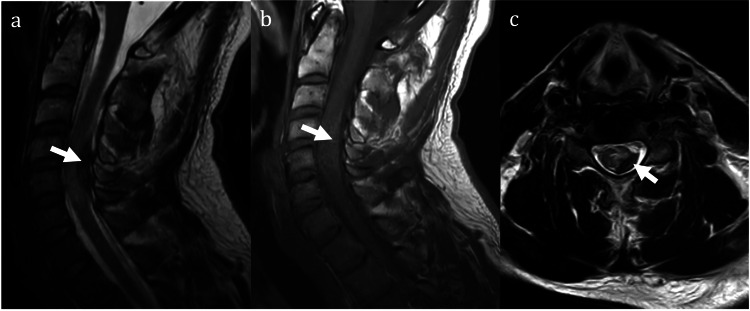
Preoperative MRI images showing traumatic spinal intradural hemorrhage The sagittal T2-weighted image shows a dorsal hypointense fusiform bleeding from C4 to C6 level with associated cord edema (a), in the sagittal T1-weighted image, the bleeding displayed isointense (b) and the axial T2-weighted image shows hypointense bleeding located in the right dorsolateral aspect of the spinal cord with central cord edema (c).
